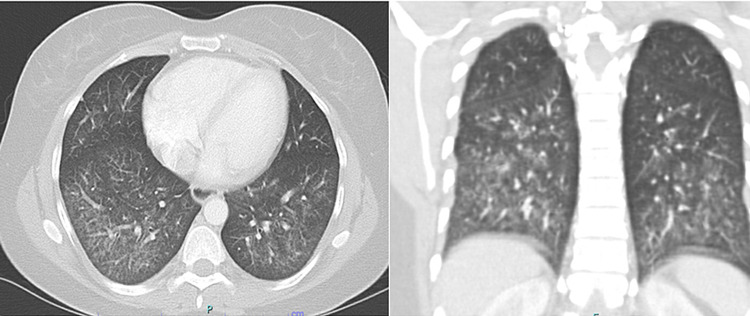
Initial high-resolution CT scan of the chest showing bilateral lower lobes predominant ground-glass opacity (coronal section on the right and a sagittal section on the left).
